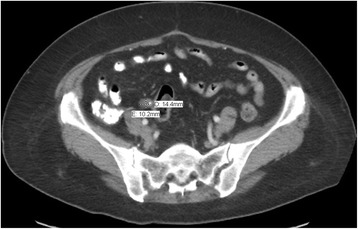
A transverse section of an abdominal CT with PO and IV contrast, revealing a mass obstructing the distal end of the appendix, measuring 14.4 mm × 10.2 mm
